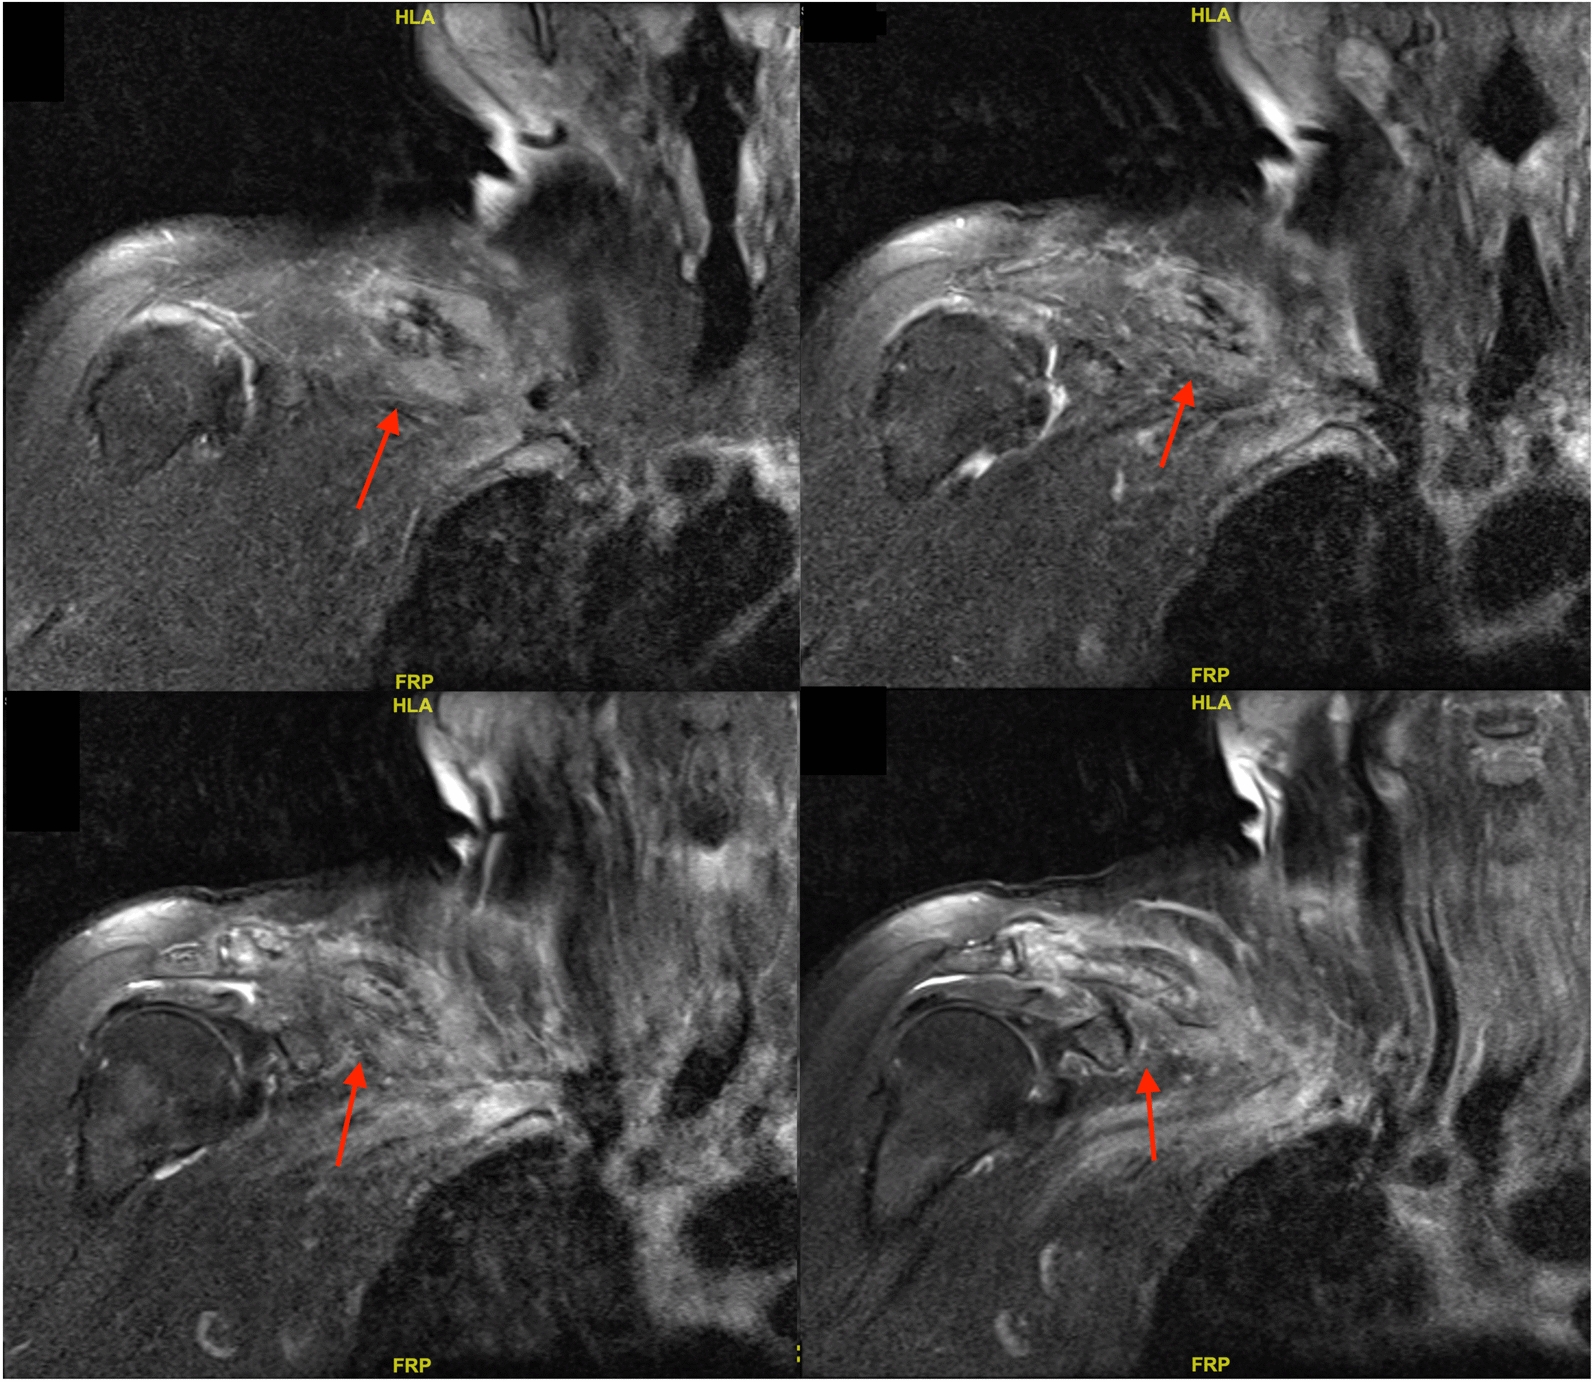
Magnetic resonance imaging (MRI) of right shoulder presented with a consistent result of an infiltrative mass lesion (9.3 cm*4.5 cm*3.3 cm, arrows) with a deconstruction of bone
